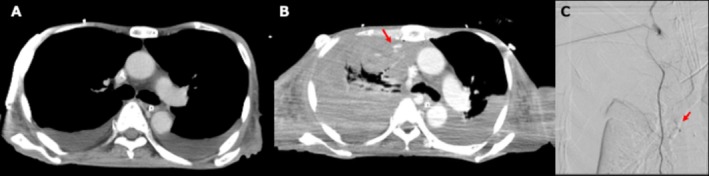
(A) Contrast‐enhanced chest CT obtained immediately after resuscitation from cardiopulmonary arrest showed only small bilateral pleural effusions, with no evidence of extravasation from the internal thoracic artery. (B) Repeat contrast‐enhanced CT performed the following day demonstrated active bleeding from the right internal thoracic artery. (C) Transarterial embolization was performed to control the active bleeding from the right internal thoracic artery.
